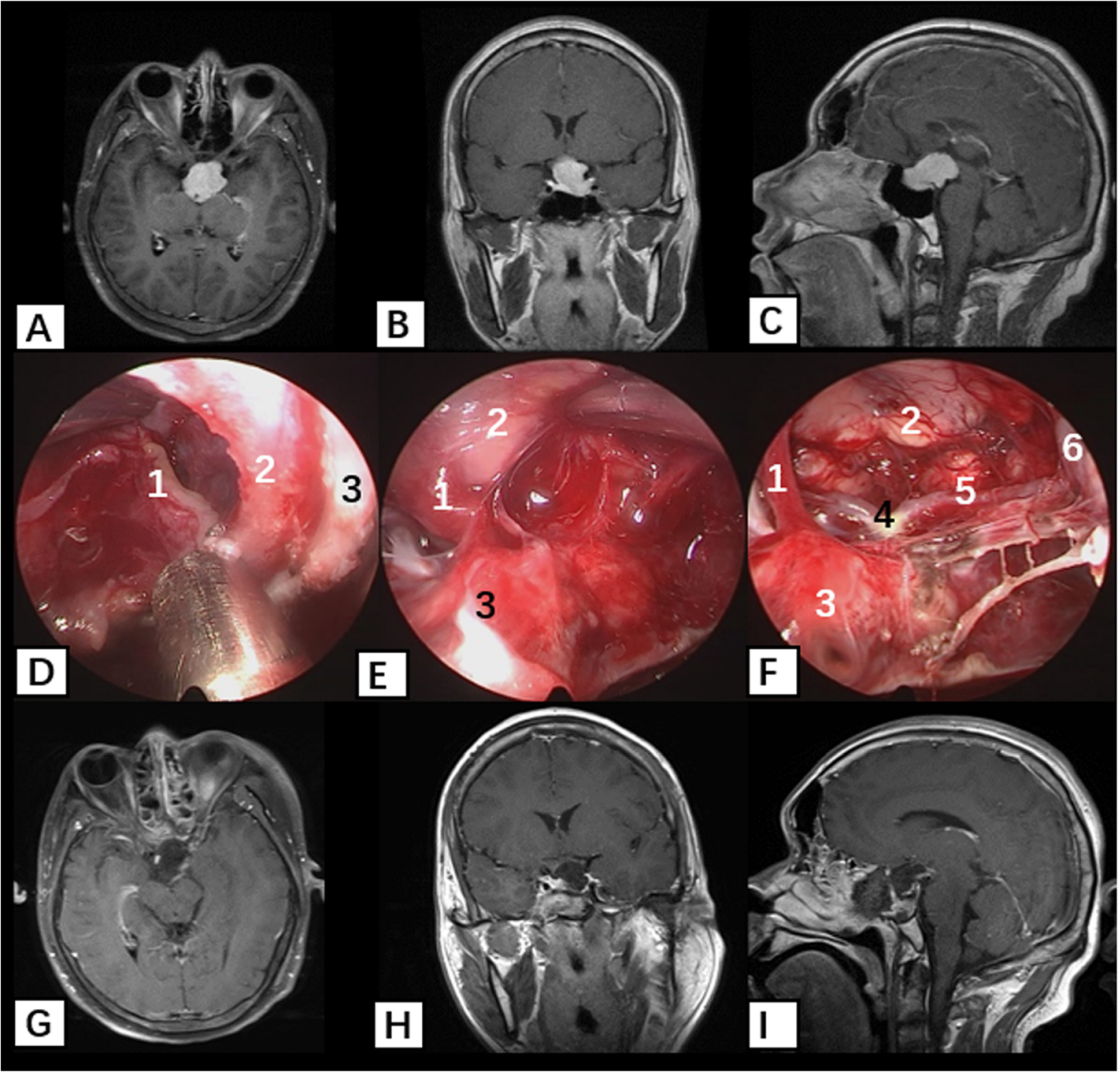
Resection of the pituitary adenomas that invade suprasellar region or clivus with endoscopic endonasal trans-sphenoidal approach. a–c Preoperative enhanced MRI T1 showed irregular abnormal signal in saddle area; d–f intraoperative findings of pituitary adenomas resection with endoscopic endonasal trans-sphenoidal approach; d resection of the intrasellar tumor, the left tumor boundary shows 1 tumor, 2 saddle dura, and 3 saddle bone; e the right tumor boundary shows 1 pituitary stalk, 2 tumor, and 3 normal pituitary; f after resection of the tumor, 1 pituitary stalk, 2 mamillary body, 3 normal pituitary, 4 base arterial bifurcation, 5 posterior communicating artery, and 6 left internal carotid artery; g–i postoperative enhanced MRI T1 showed total tumor resection
